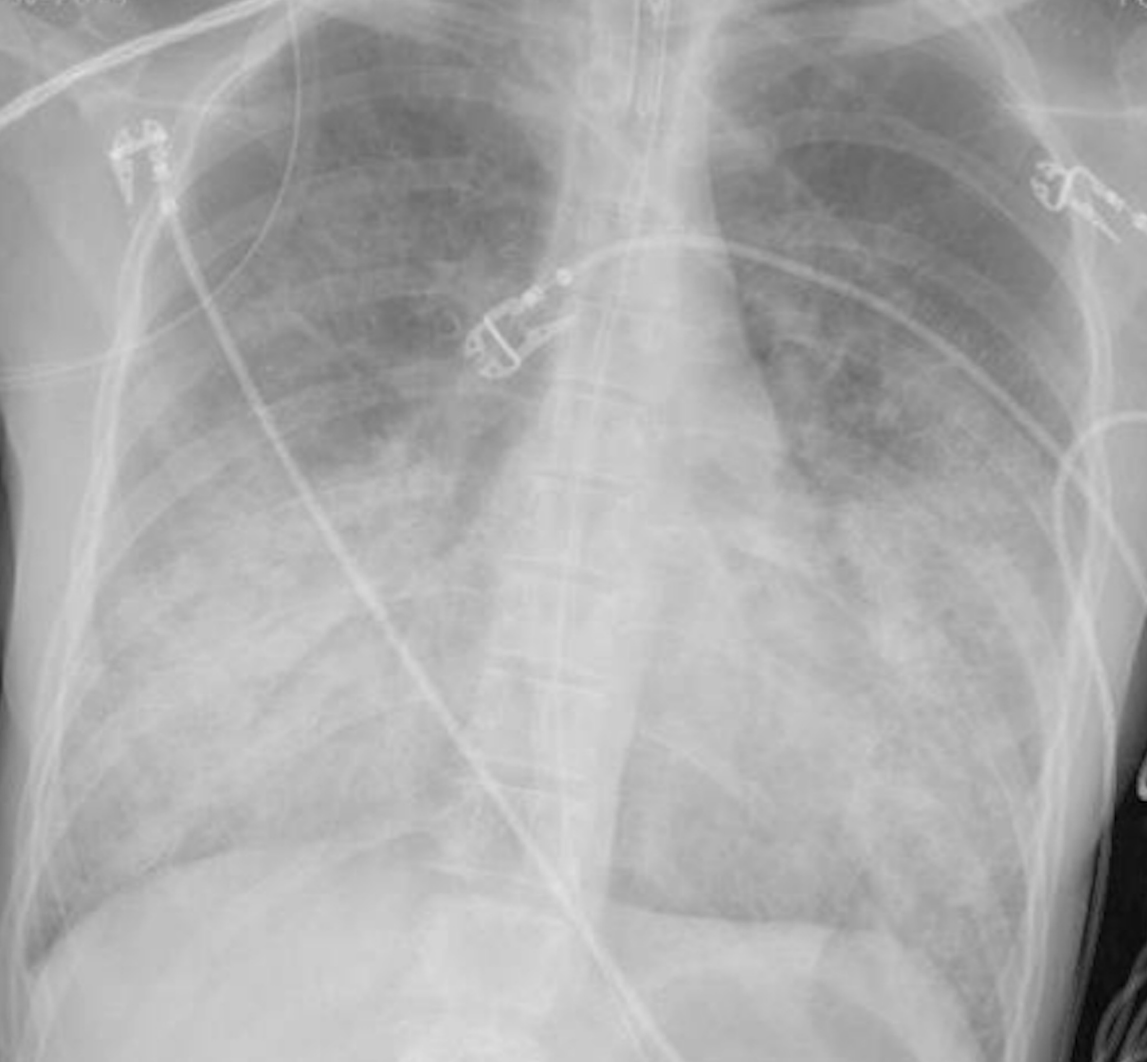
Chest X-ray on admission The image shows confluent areas of consolidation in the bilateral mid to lower lung zones
